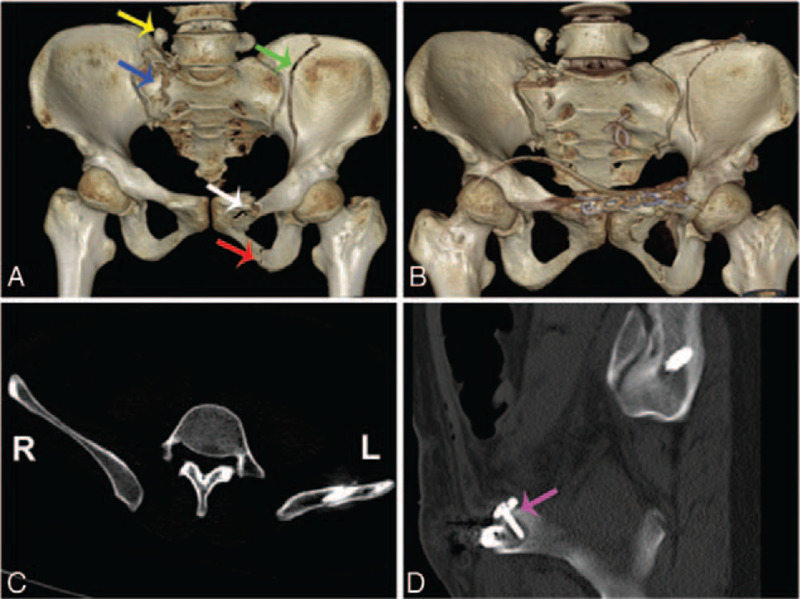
(A) Preoperative 3D CT showed that there was bone discontinuity and displacement in the left superior (the white arrow) and inferior (the red arrow) pubic ramus, and a gap was showed though the ilium (the green arrow). The sacrum (the blue arrow) was not in regular shape and the right transverse process of the L5 vertebral (the yellow arrow) was also found to be irregular. (B) Postoperative 3D CT scanning. (C) Postoperative CT image showed that anterior inferior iliac spine screw was through the fracture line and entirely in cancellous bone. (D) Postoperative CT image revealed that the iliac screw was in good position (the purple arrow). 3D = three dimensional, CT = computed tomography.
